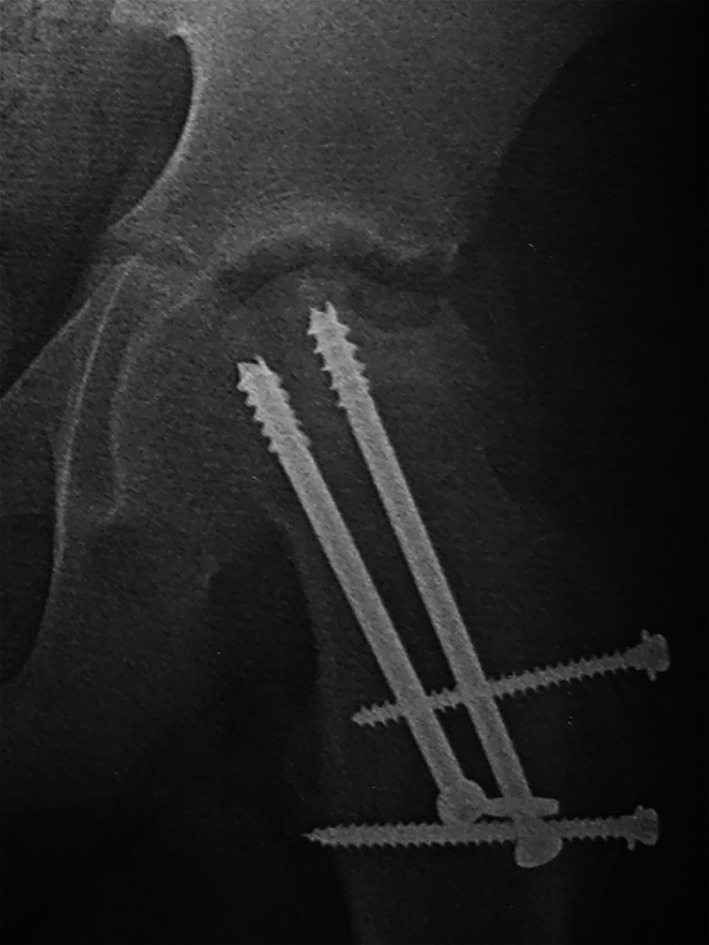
Plain X-ray (AP view) showing segmental avascular necrosis of the femoral head
